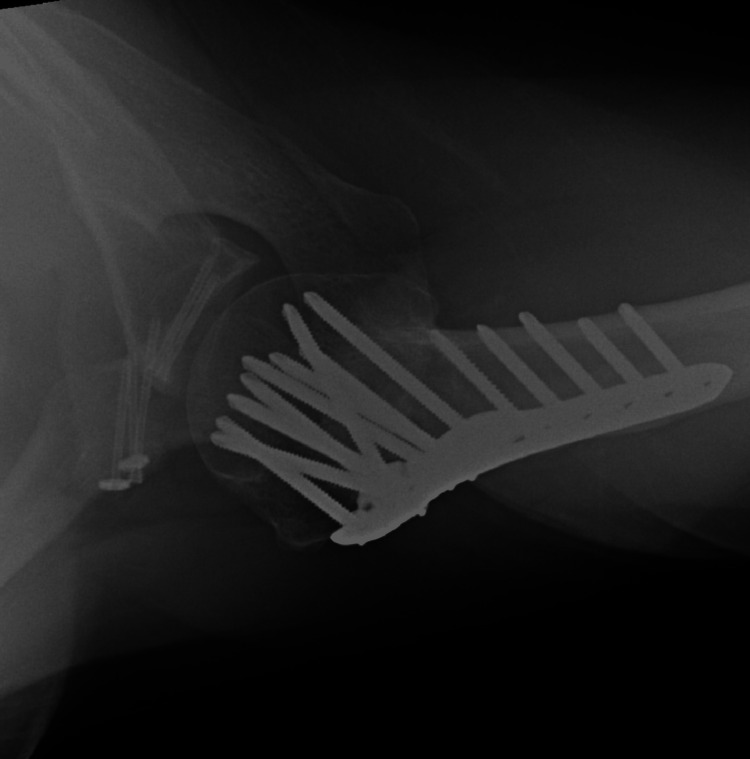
Postoperative axial radiograph at 14 months after surgery The union of all three fractures is evident, and the glenohumeral joint remains concentric.
